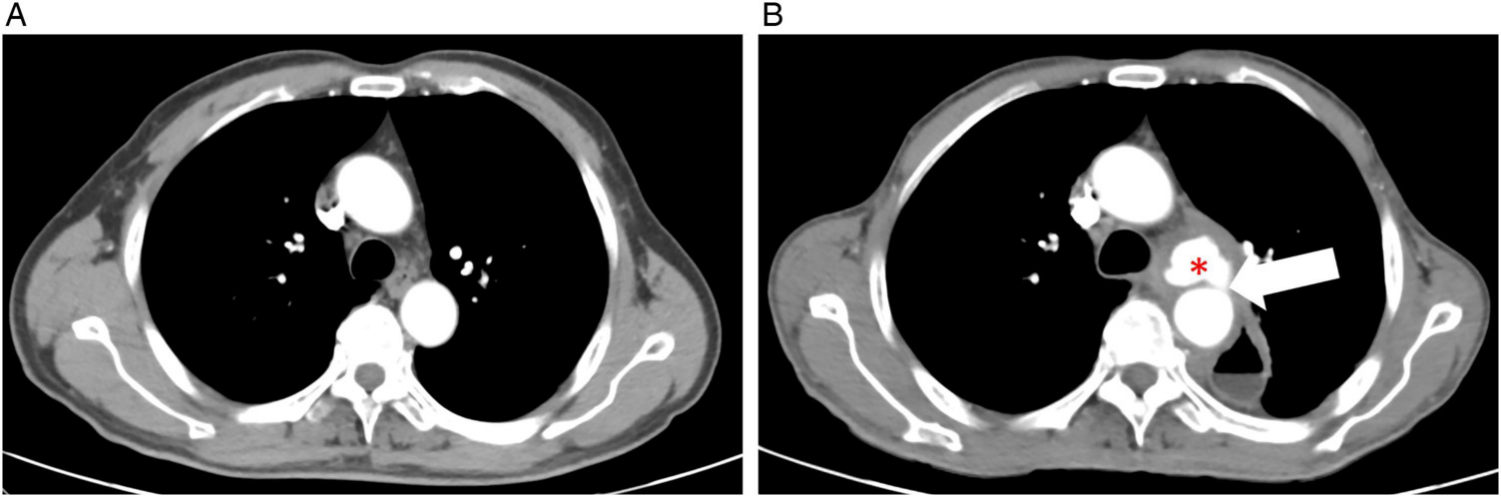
Contrast-enhanced computed tomography (CT) of the chest reveals the aortic injury. (A) Axial view from a pre-dilation contrast-enhanced CT (May 8, 2023), the radiological report of which described aortic wall calcification (indicating pre-existing atherosclerosis). (B) Axial view from a post-dilation contrast-enhanced CT (November 9, 2023) showing a penetrating aortic ulcer (PAU) with contained rupture (indicated by the white arrow), evidenced by a focal collection of contrast outside the lumen, and a surrounding hematoma (indicated by the asterisk).
